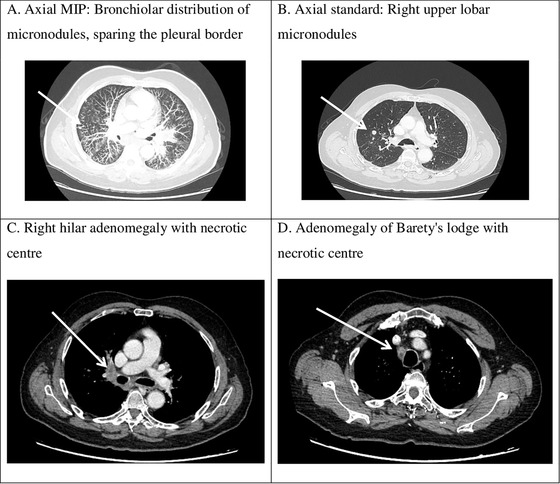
Chest CT scan sections revealing active pulmonary tuberculosis
